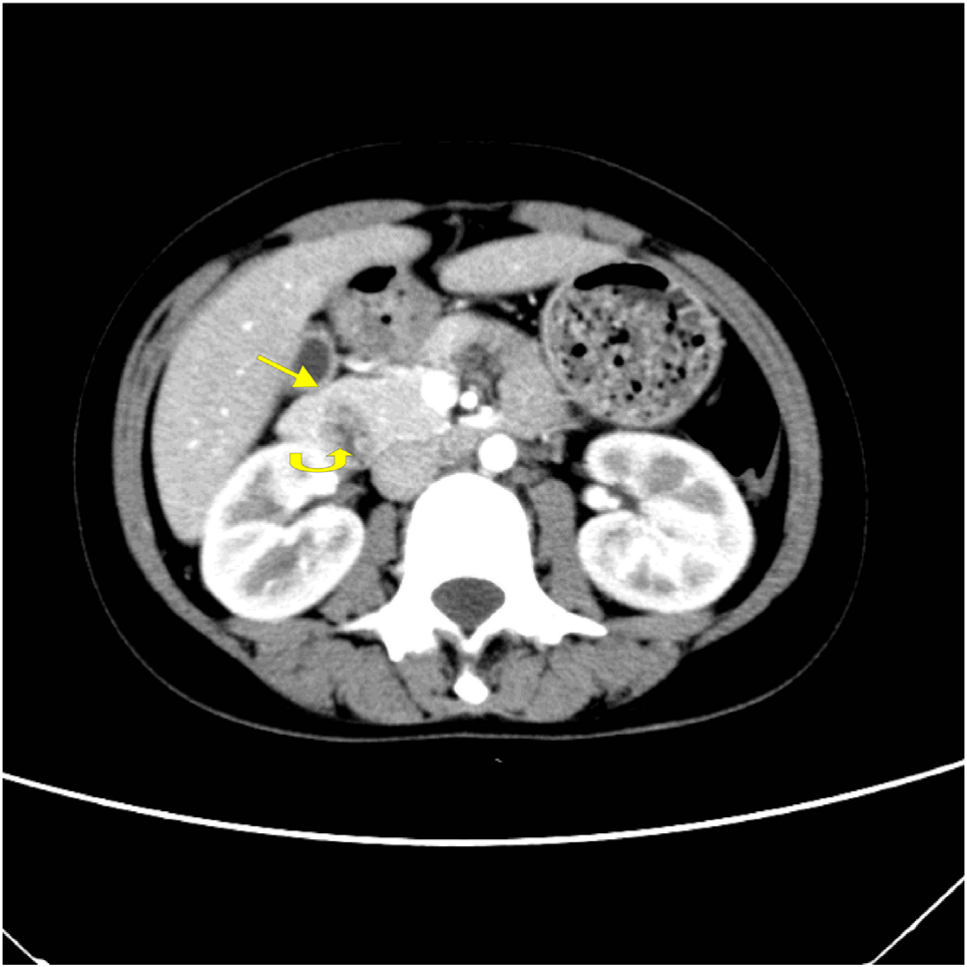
A 22‐year‐old woman with abdominal pain. Axial multiple detector spiral computed tomography (MDCT) venous phase shows pancreatic tissue (arrow) extending in an anterolateral direction towards the duodenum (curved arrow)
